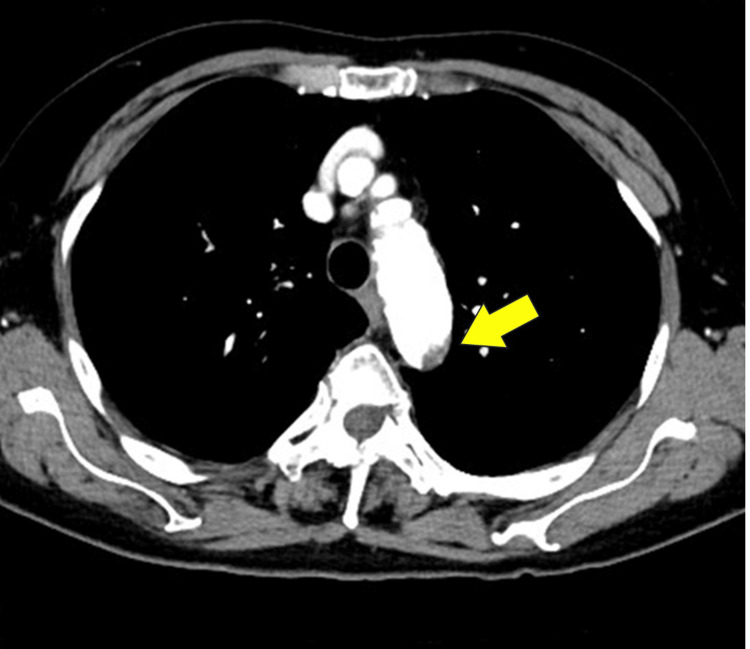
Preoperative enhanced CT of the patient’s aortic arch The arch aorta is slightly shaggy, and a plaque in the wall (arrow) is seen on the arch aorta’s greater fold side.
